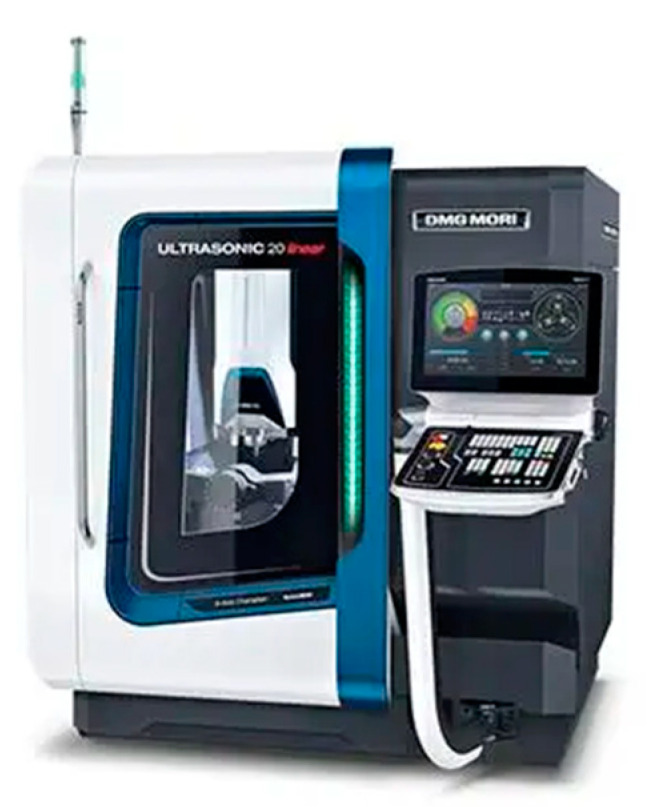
DMG Ultrasonic 20 linear high-speed machining center.
